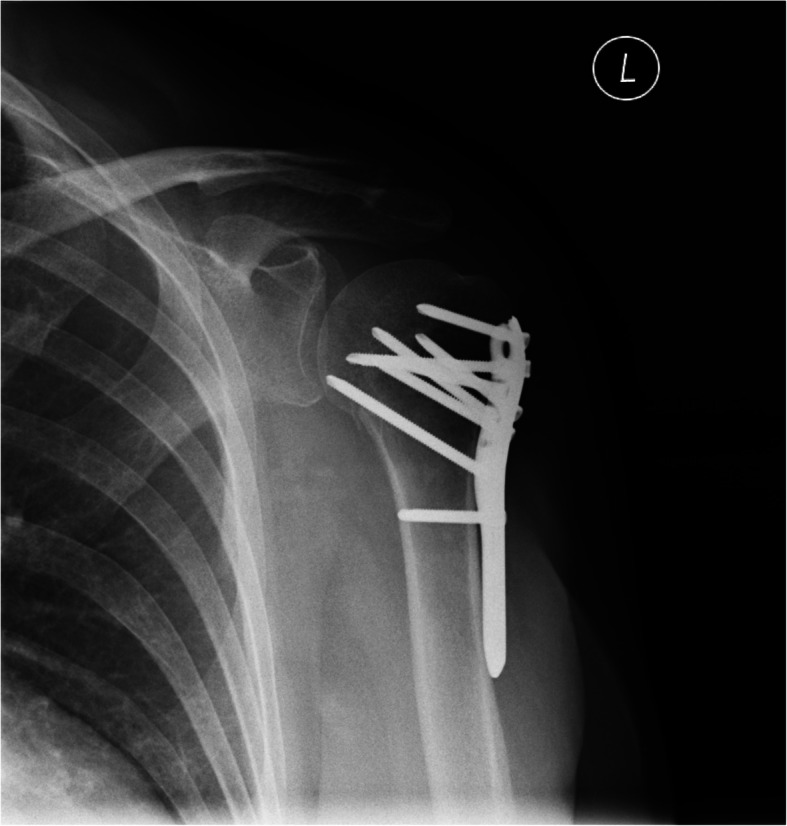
Philos plate before removal (X-ray)
